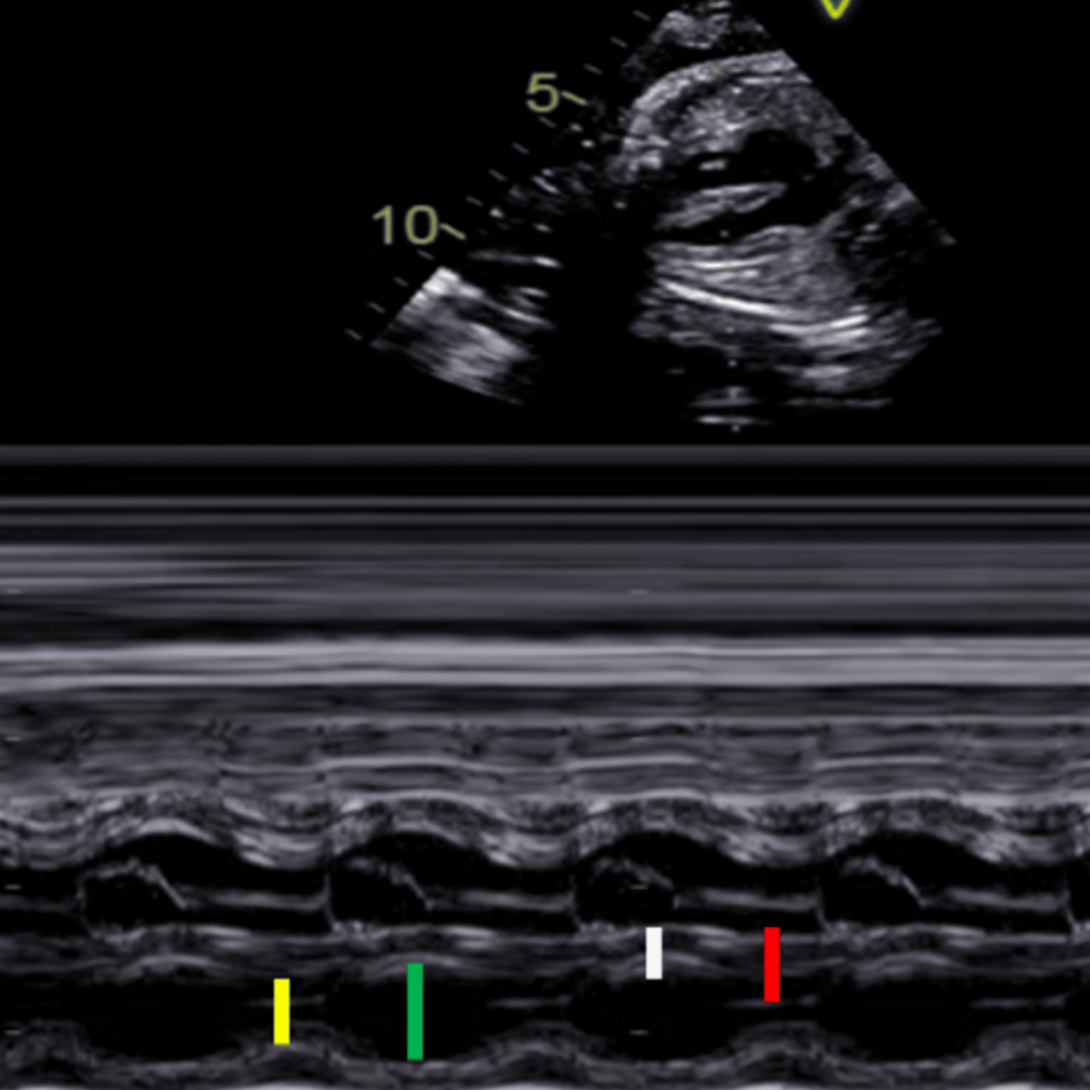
M-mode echocardiographic image obtained from the fetal four-chamber view demonstrating measurement of interventricular septal thickness during diastole (IVSd) and systole (IVSs), as well as left ventricular internal dimensions during diastole (LVIDd) and systole (LVIDs). The white line represents IVSd. The red line shows IVSs. The yellow line marks the LVIDs, and the green line indicates the LVIDd.
